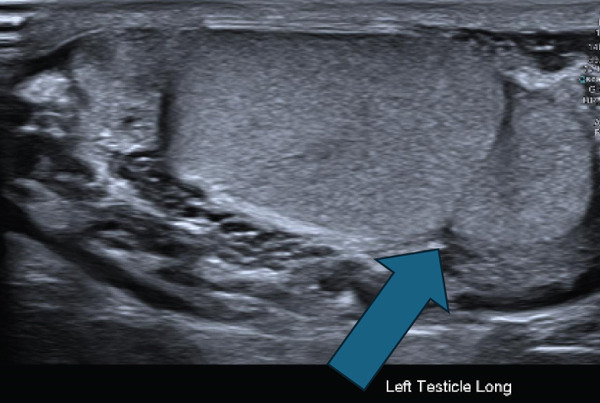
A follow‐up ultrasound performed 18 months later demonstrated no change, continuing to display identical echotexture, supporting the diagnosis of a bilobed testis. Arrow demonstrating ridge between each lobe of the left testicle.
